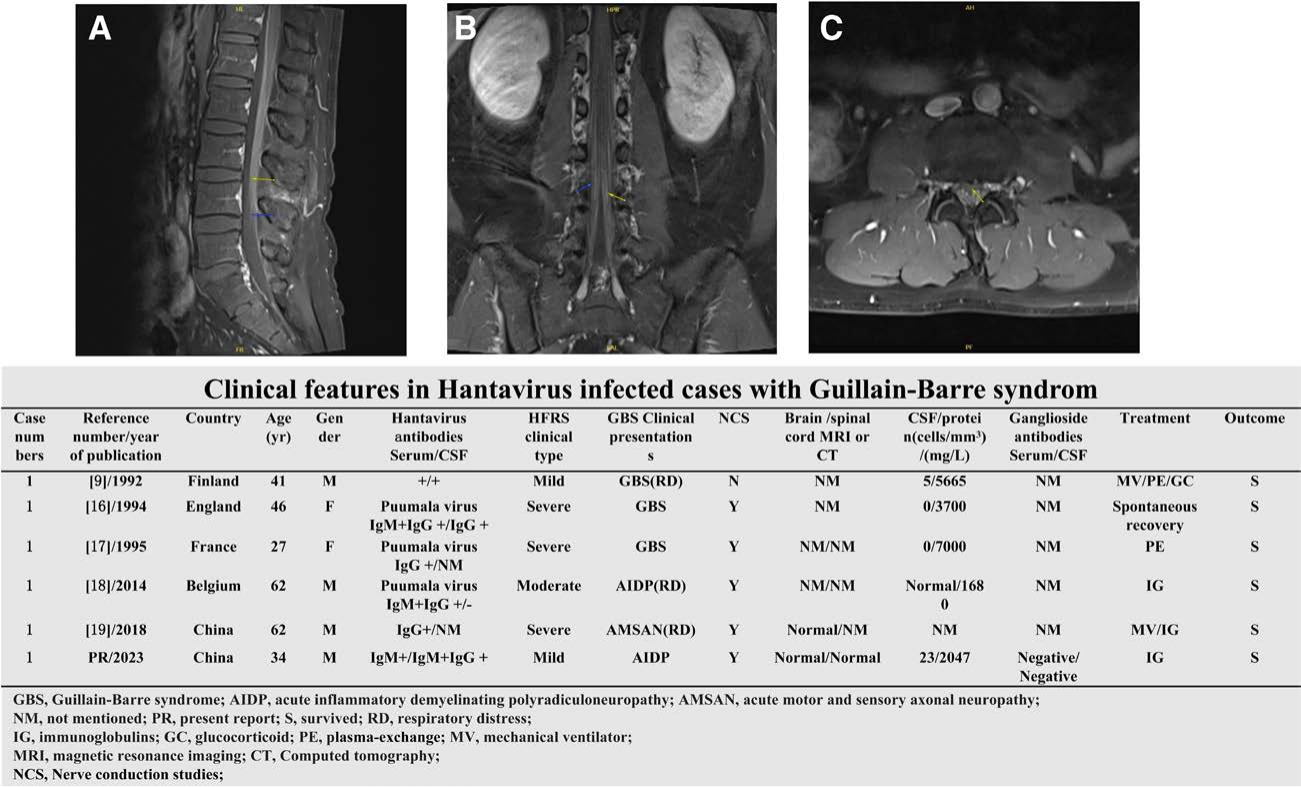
Gadolinium-enhanced MRI of lumbar segment of spinal cord of this case and clinical features in Hantavirus infected cases with Guillain–Barre syndrome. The arrows showed the linear enhancement at the nerve root—(A) sagitta, (B) coronal, and (C) transverse view.
